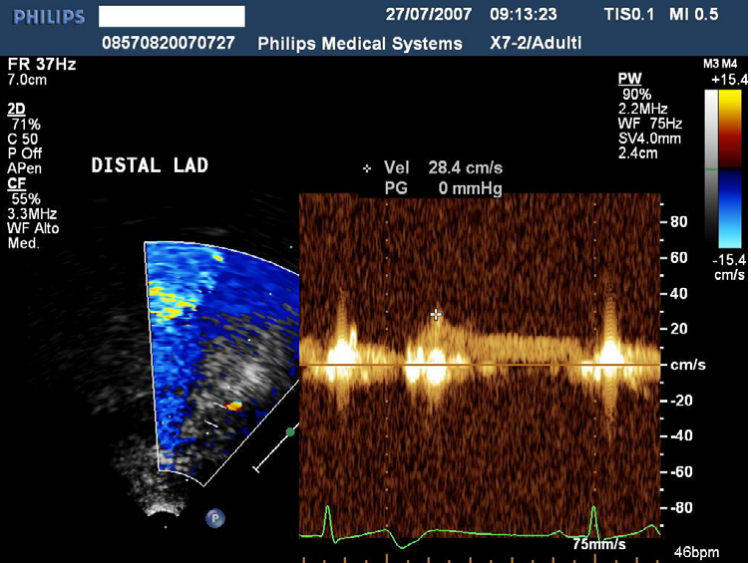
shows the Pulse Doppler envelope of distal tract of left descending coronary artery.
